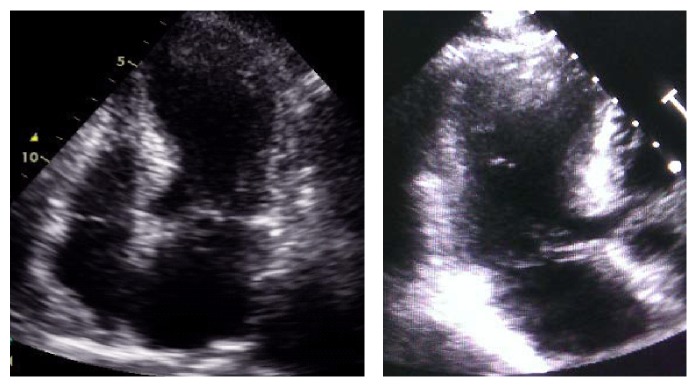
Echocardiography showing apical hypokinesis with preserved basal function.
